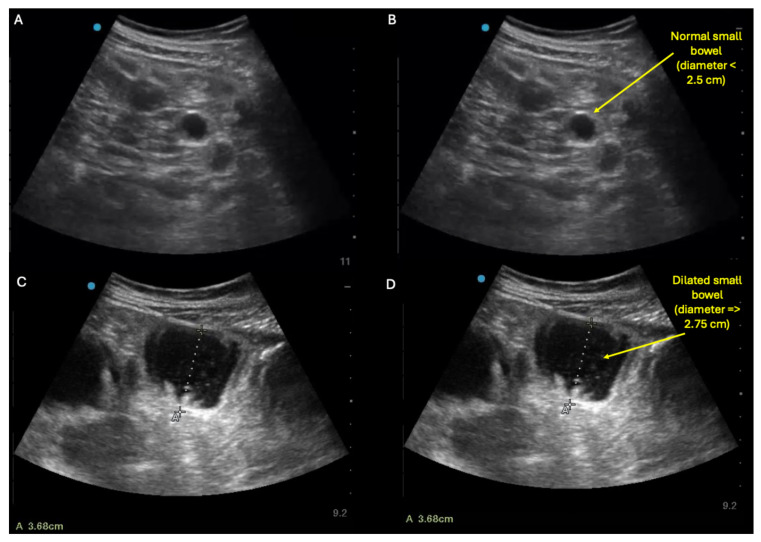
Similarly to small bowel ileus, small bowel obstruction (SBO) on ultrasound will appear as dilated loops of bowel, defined as small bowel diameter ≥ 2.75 cm [11,12,13]. But in contrast to ileus, SBO may also show decompressed small bowel loops in the same abdomen. Thus, the characteristic sonographic finding of small bowel obstruction is concurrent visualization in the same abdomen of both (a) small, decompressed (≤2.5 cm diameter) loops of small bowel (top panel: A,B) and (b) dilated (≥2.75 cm diameter) loops of small bowel lacking peristalsis (bottom panel: C,D) (see also Supplementary Video S4 for examples of these dynamic findings). However, in some cases of SBO, the decompressed loops may not be visualizable with ultrasound, making it difficult to differentiate SBO versus ileus using ultrasound alone. In such cases where ultrasound reveals diffusely dilated loops of small bowel with no decompressed loops and SBO is still suspected, adjunctive imaging such as X-ray and/or CT scan can be pursued.
